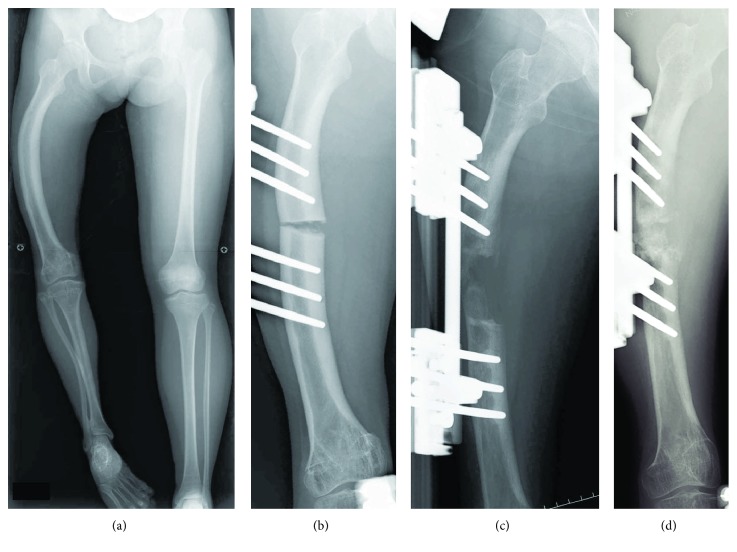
Radiographs of the lower extremities at the time of the second lengthening. (a) An anteroposterior supine radiograph of the lower extremities at eleven years of age before the second lengthening revealing LLD of 11 cm. (b) A radiograph of the right femur just after the femoral osteotomy demonstrating acute correction of an anterolateral bowing deformity. (c, d) Radiographs of the right femur before (c) and after (d) the chipping surgery.
